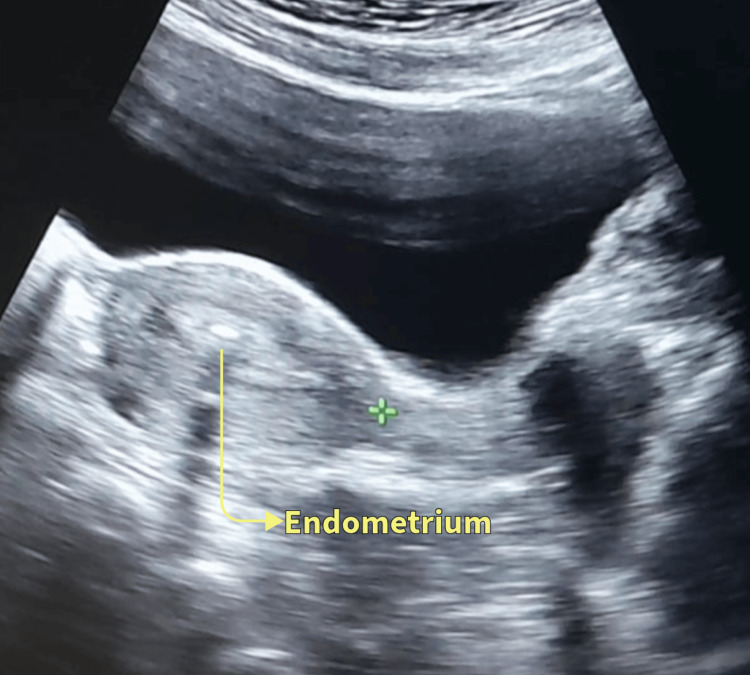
Ultrasound sonography of the female patient on day 17 of menstrual cycle
